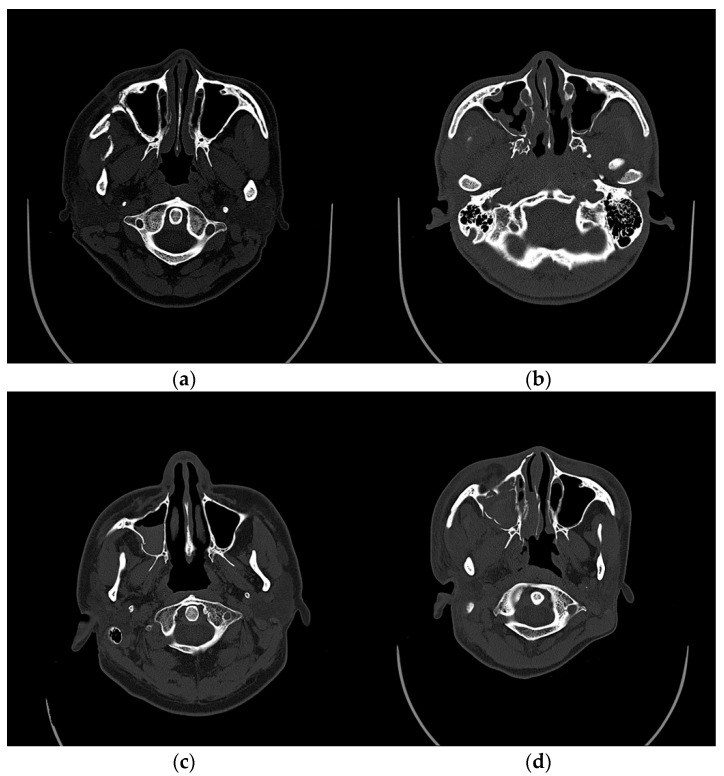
Representative CT sections showing the different preoperative evaluations of the maxillary sinus. (a) normal; (b) maxillary mucosal thickening; (c) gas–fluid level; (d) total opacification.
